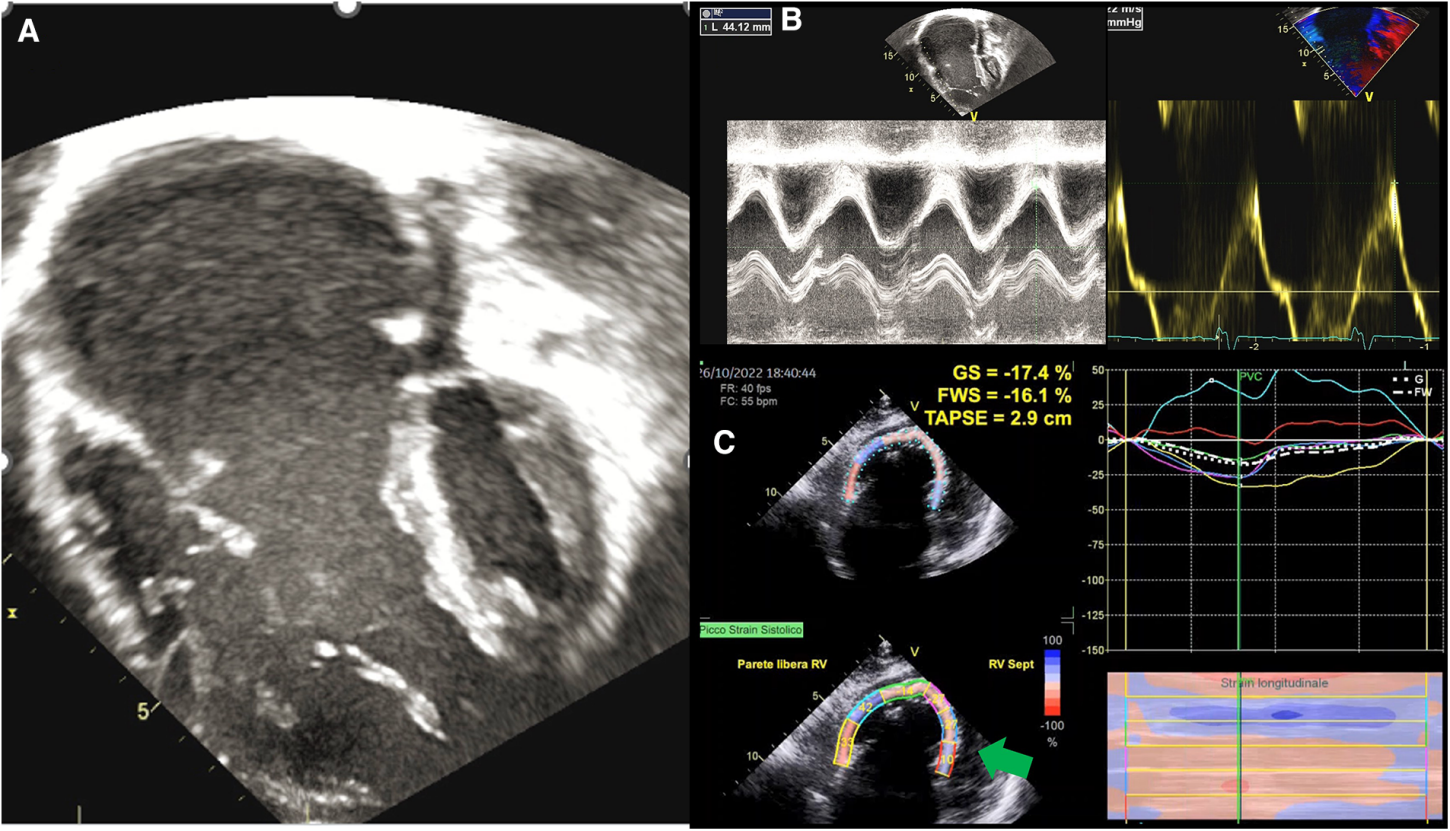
Functional assessment in ebstein anomaly. (A) EA as visualized by 2DE; (B) standard echocardiographic parameters to assess RV function, such as TAPSE an S’ wave, are increased in EA because of volume overload; (C) GLS analysis shows that the RV in EA is “atrialised” not only morphologically, but also functionally, as basal and medial septal strain values (green arrows) are positive in systole, thus behaving as an atrium. EA, ebstein anomaly; 2DE, two-dimensional echocardiography; TAPSE, tricuspid annular plane systolic excursion; GLS, global longitudinal strain; RV, right ventricle.
